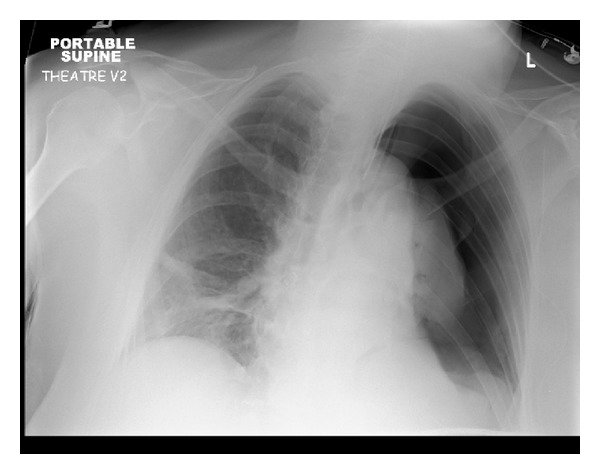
Chest X-ray taken ten minutes after partial decompression of left sided tension pneumothorax (patient still requiring positive pressure ventilation).
